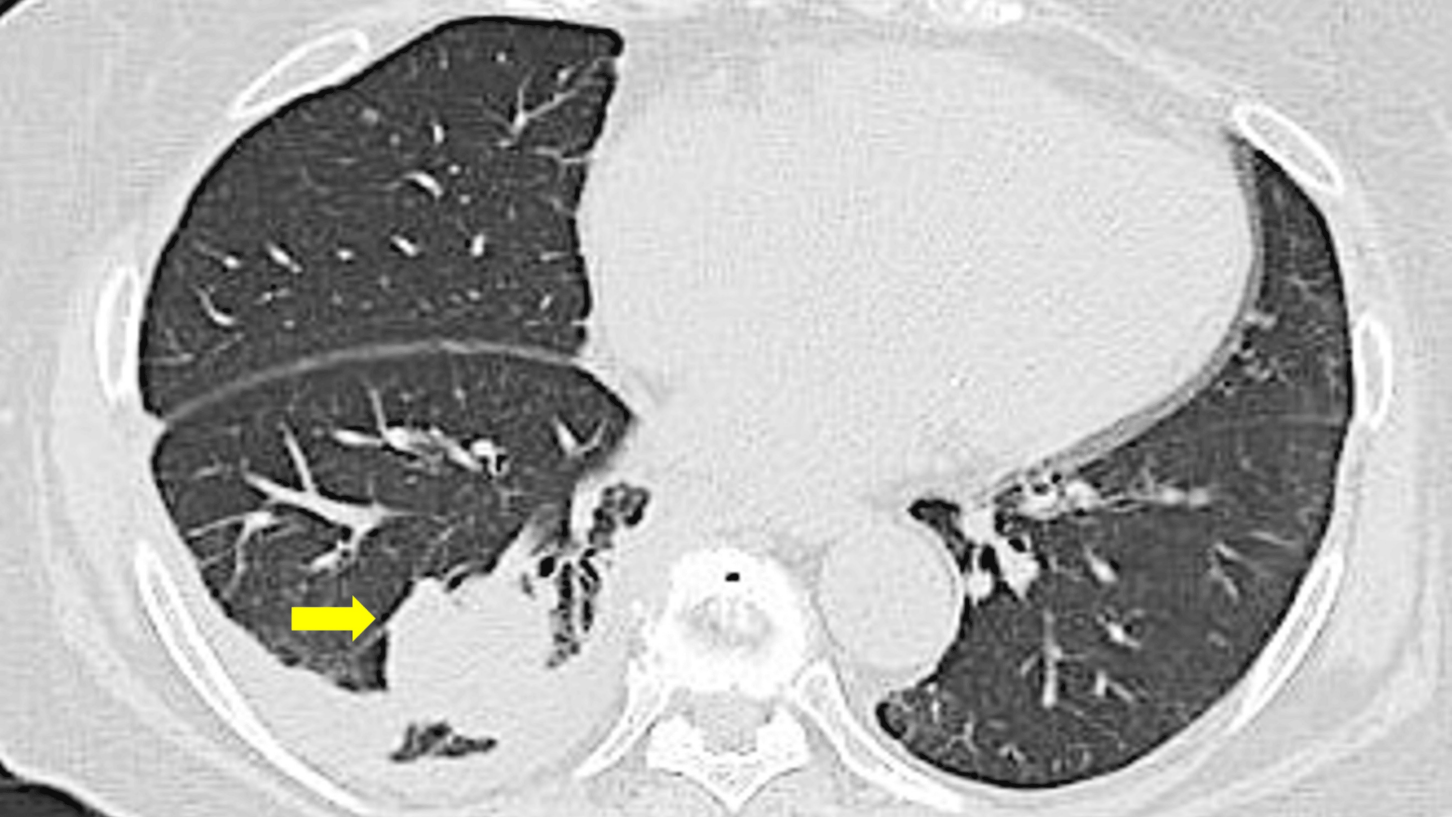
Chest computed tomography scan shows a 30-mm mass in the right lower lobe (arrow).
